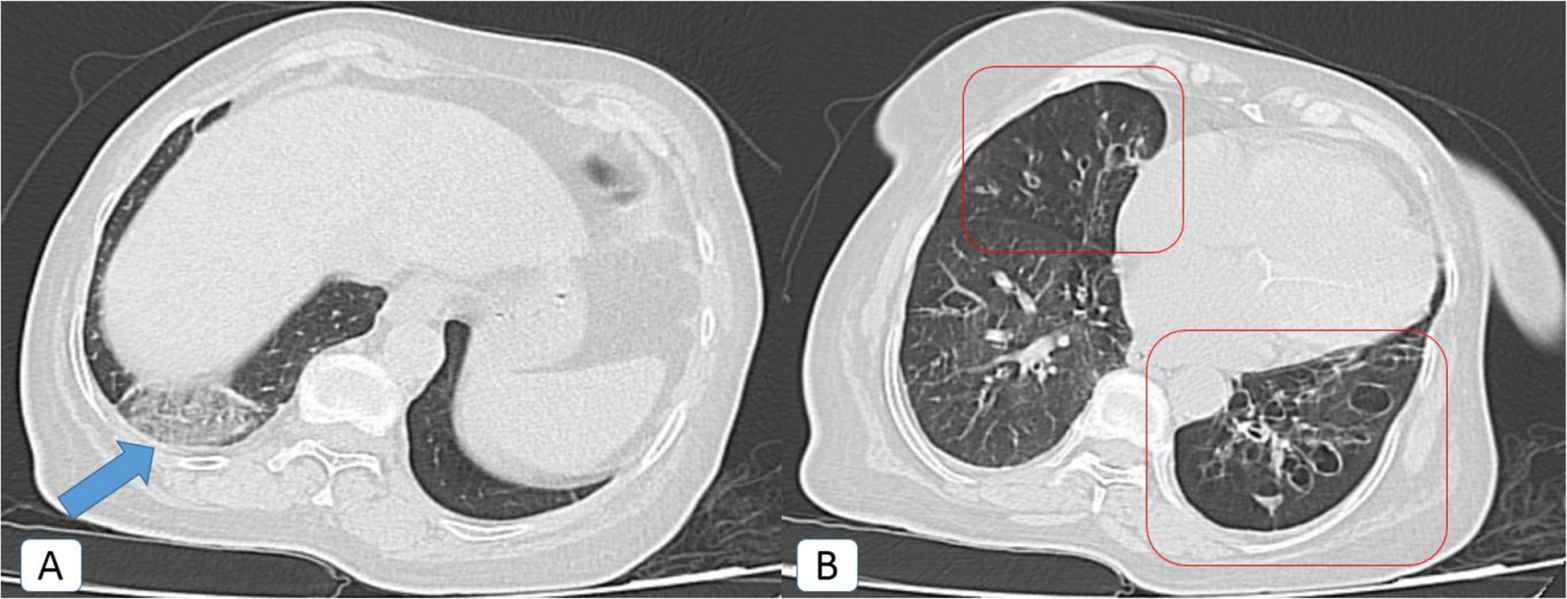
COVID-19 infection atypically presented by cystic bronchiectasis: a 58-year-old female COVID-19 patient without a history of previous chest disease. A Axial chest CT (lung window) showing right basal sub-pleural ground glass patch (blue arrow). B Axial chest CT (lung window) showing right middle lobar traction bronchiectatic changes and left lower lobar cystic bronchiectatic changes with air-fluid levelling and air trapping (red squares)
